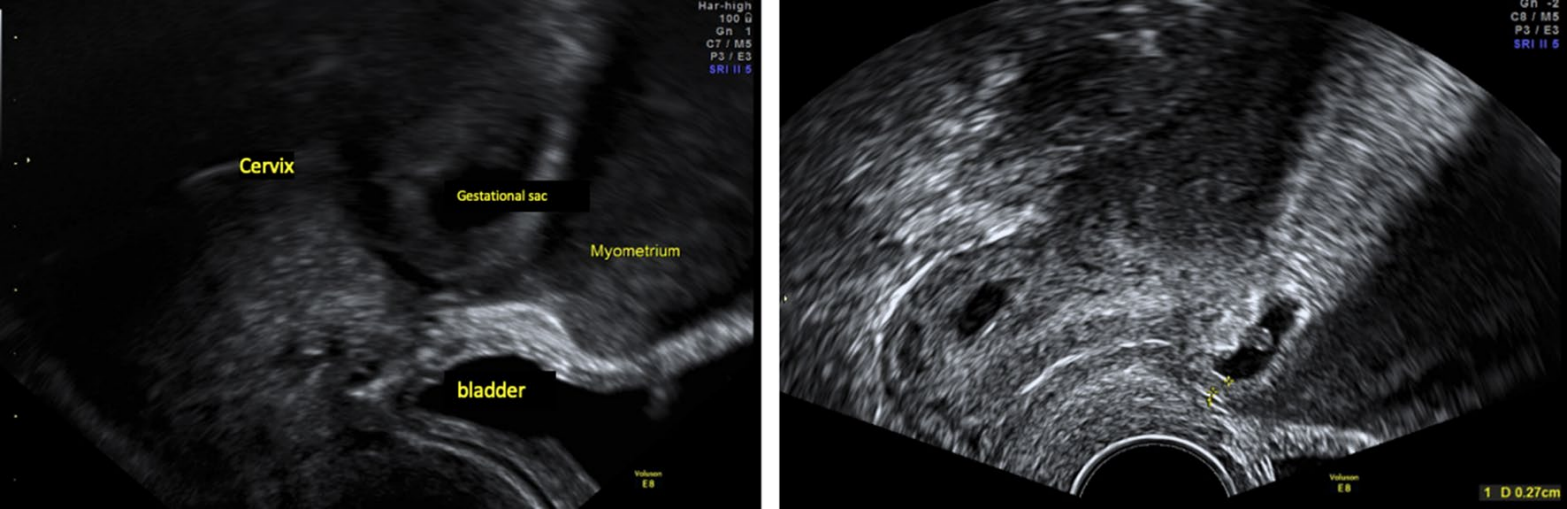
Ultrasound of CSP in a 42-year-old woman (G3 P2) in 6^2/7^ weeks of gestation, with one prior C-Section and a uterine wall remaining 0.27 cm
